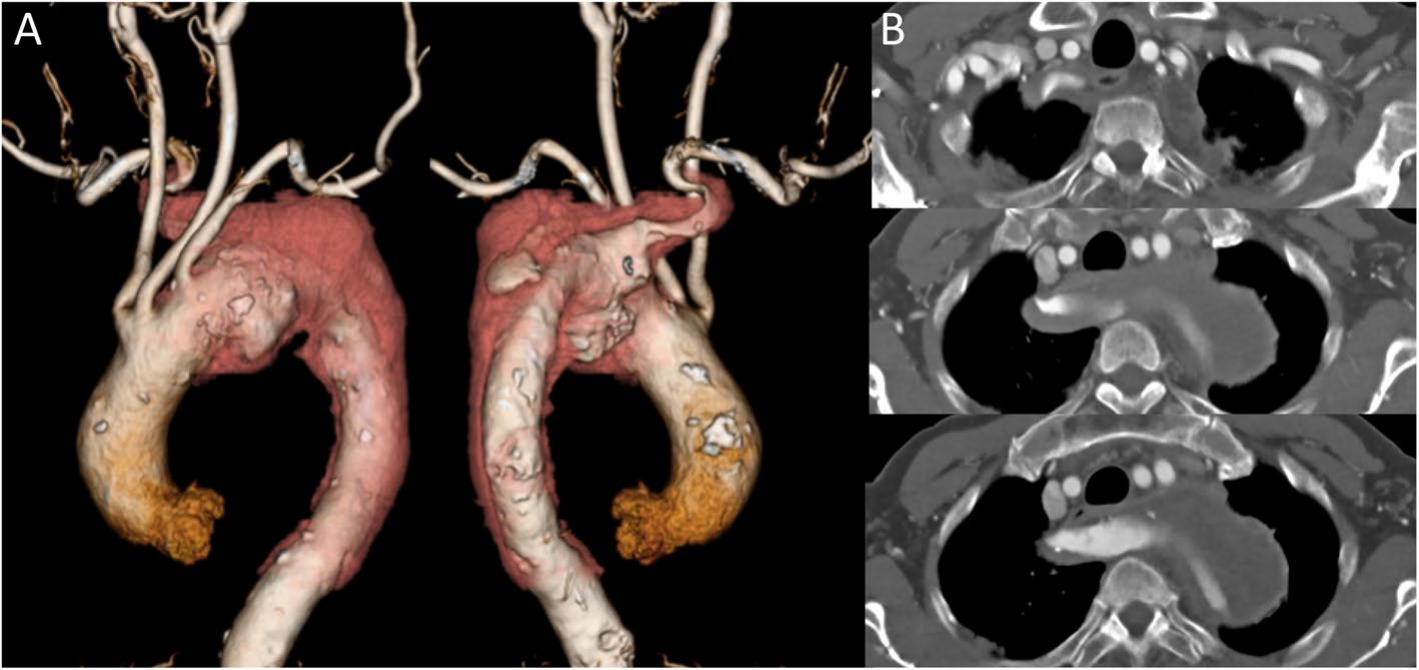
Preoperative computer tomography (CT). A Three-dimensional CT images (anterior and posterior). Distal aortic arch aneurysm accompanied by a Kommerell diverticulum which developed aortic dissection. The aberrant right subclavian artery (ARSA) was remarkably bent at approximately 180° twice. B Axial images showed that the esophagus was compressed between the trachea and the enlarged ARSA
